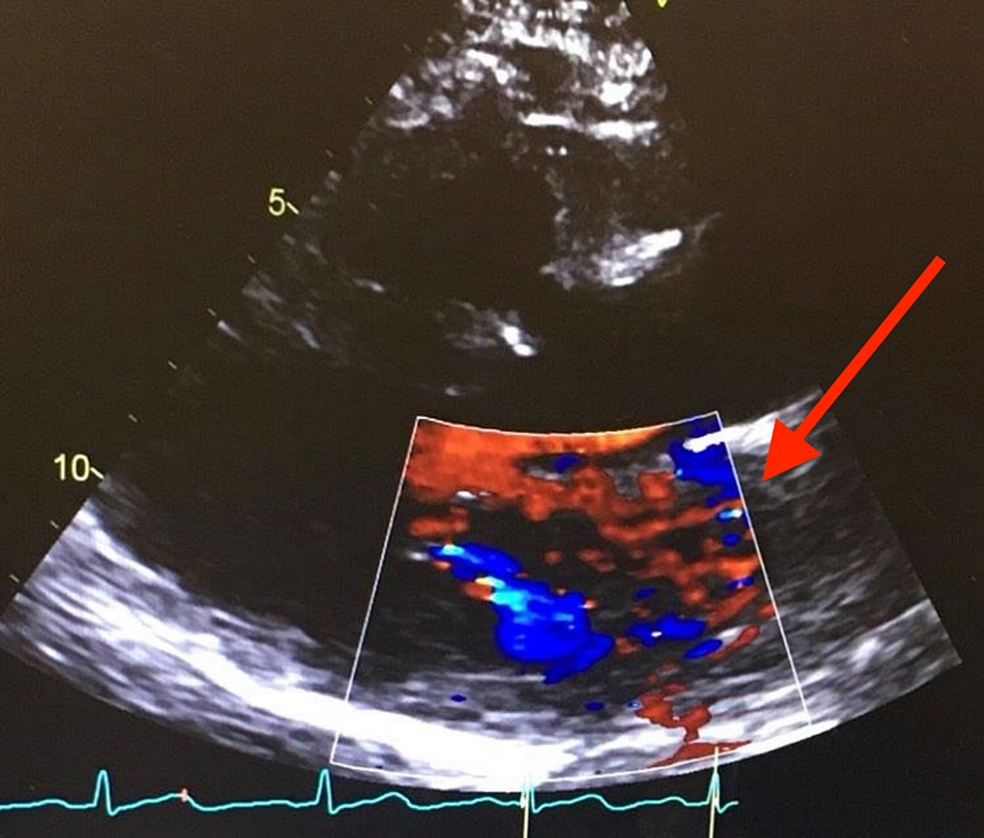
Parasternal long-axis view of echocardiogram showing severe mitral regurgitation.
